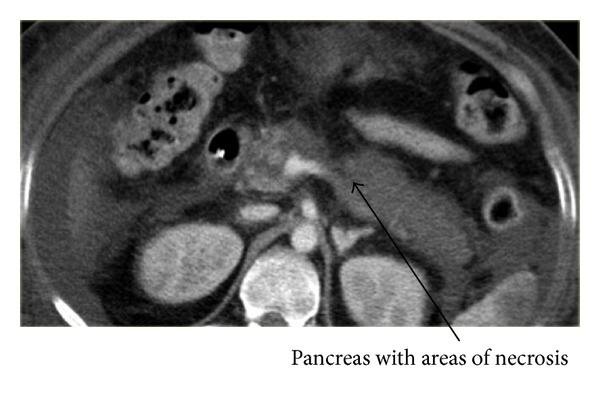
Representative CT scan of severe acute pancreatitis. CT scan with oral and intravenous contrast: >50% of the pancreas does not enhance consistent with necrotizing pancreatitis.
